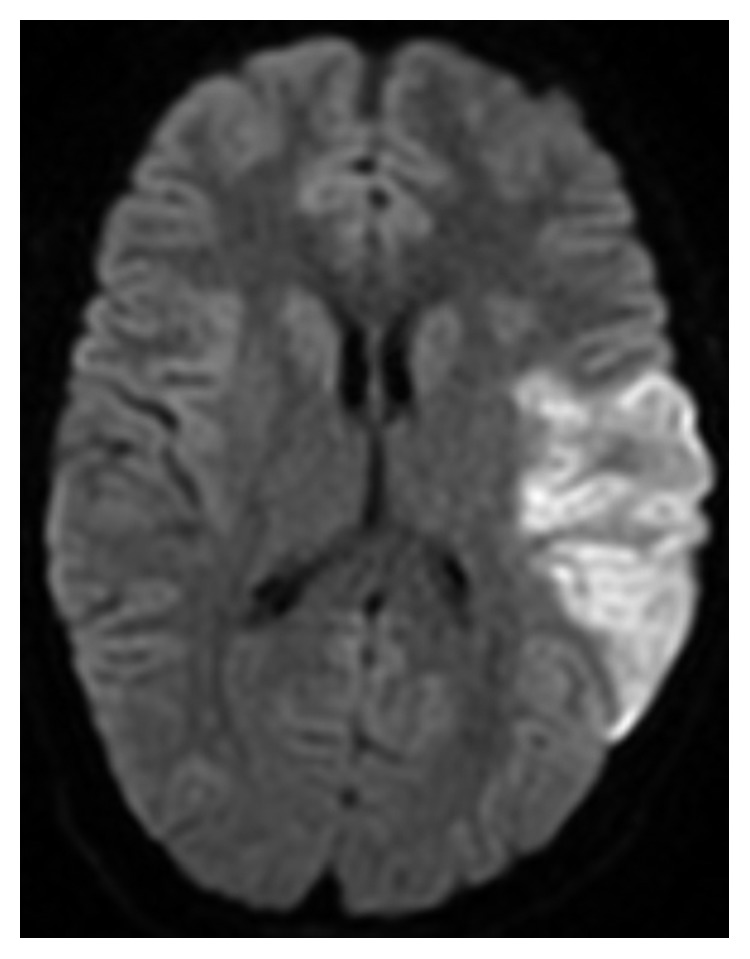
MRI brain without contrast (DWI—diffusion-weighted image) showing acute ischemic infarct in left temporoparietal lobe in the distribution of left MCA.
